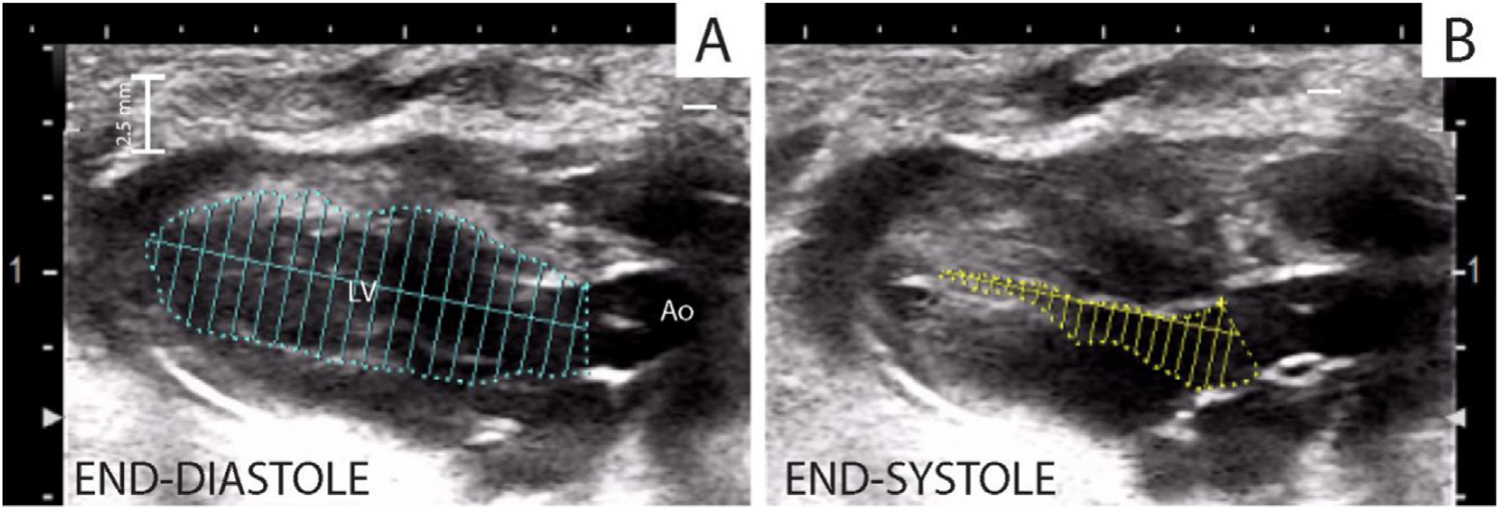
Left ventricular (LV) volumes, end-diastolic volume EDV (A), end-systolic volume ESV (B) and LV ejection fraction (EF) were calculated by the modified simple plane Simpson’s rule from the parasternal long-axis view.
